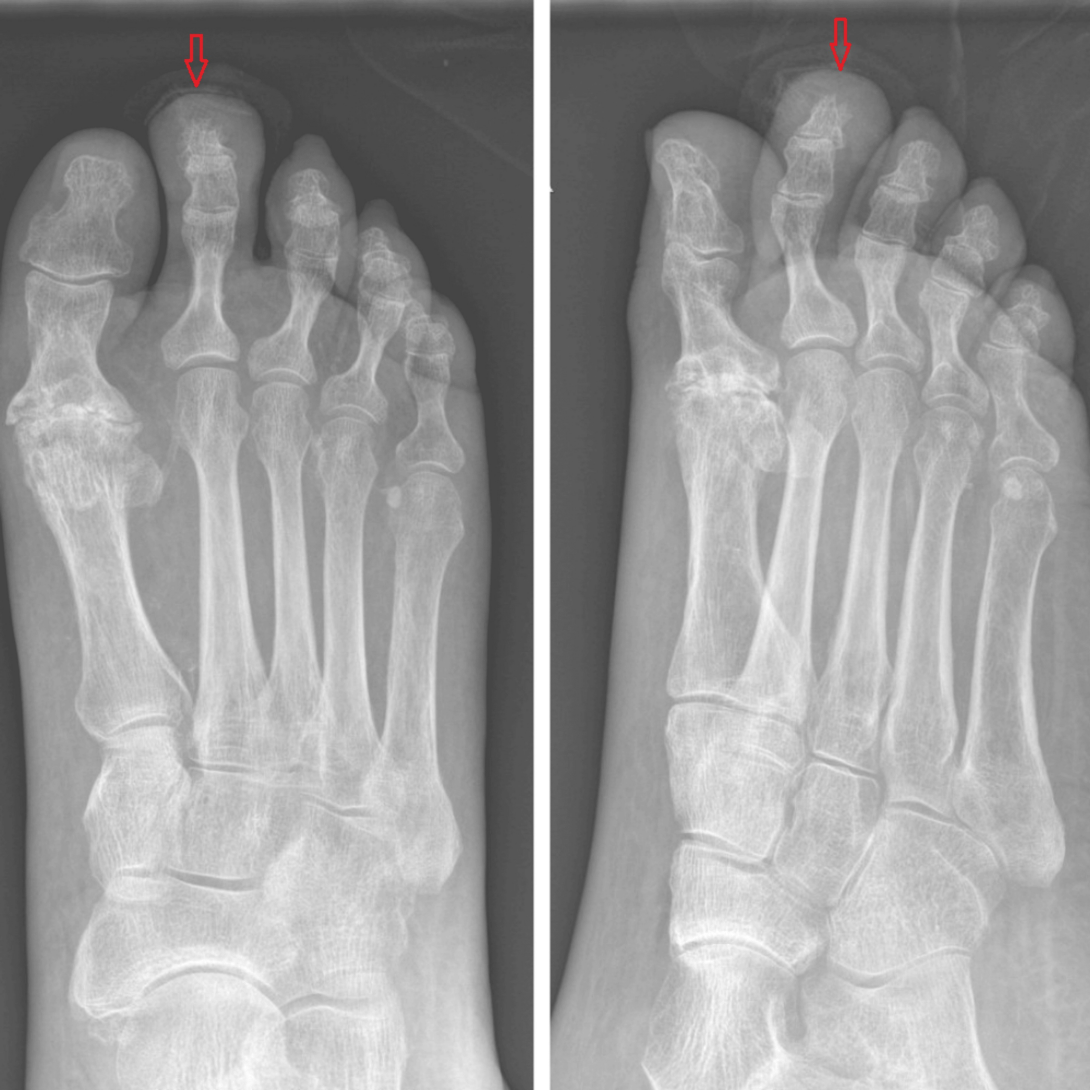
Right-foot X-ray - AP and lateral views Xray Right foot showed irregularity of the terminal tuft of the distal phalanx of second toe with several cortical erosions; significant surrounding soft tissue swelling noted, findings suggestive of osteomyelitis.
